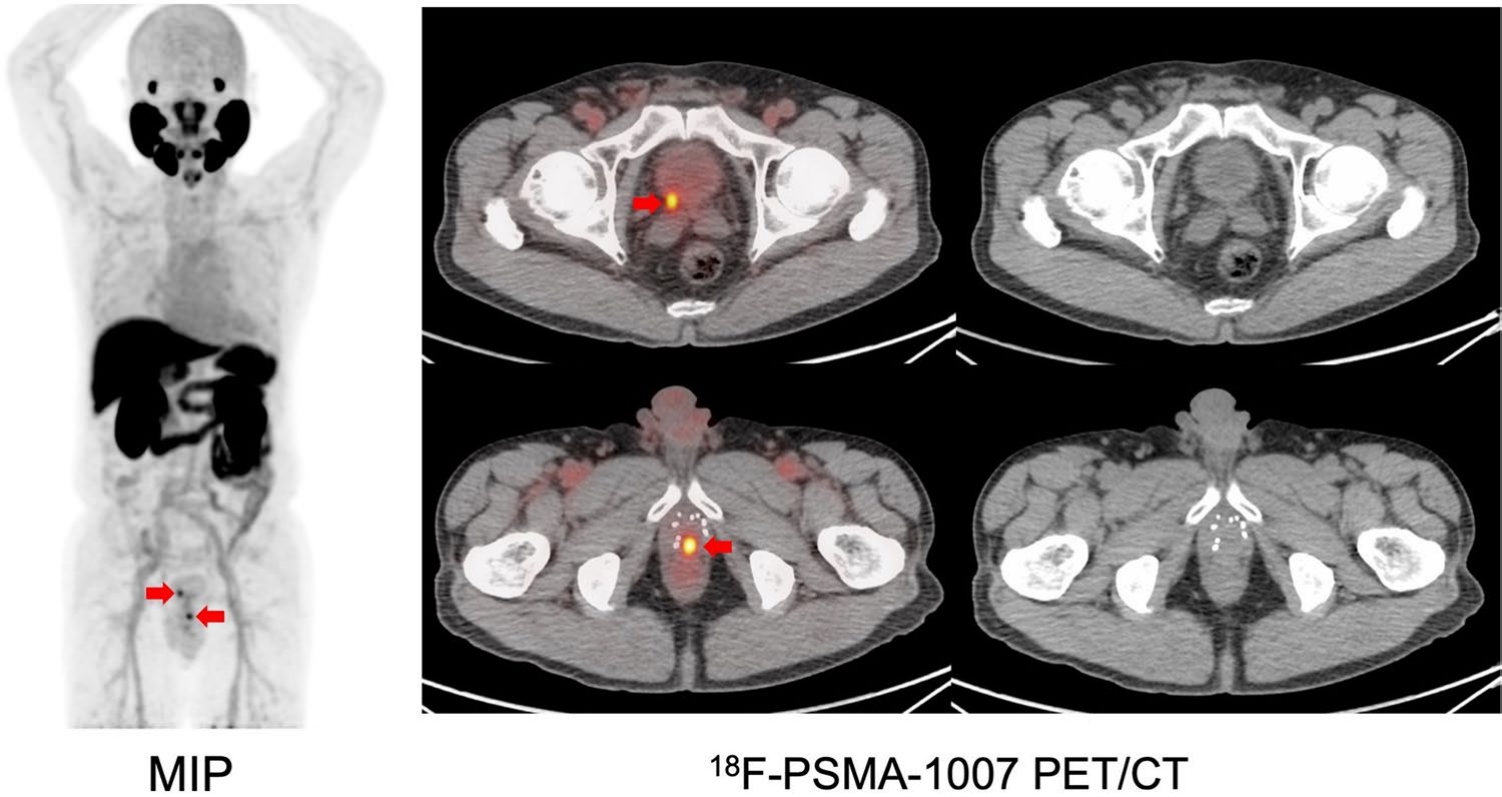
[^18^F]PSMA-1007 PET in BCR patient after ^125^I-seed implantation (PSA at PET: 3.32 ng/mL) (MIP: maximum intensity projection). Focal uptakes are observed on PSMA-PET (red arrows) and biopsy on the caudal lesion revealed a recurrence. Radiation therapy (cyber-knife) is performed targeting the two lesions and PSA value shows a decrease (PSA at 7 months after cyber-knife: 0.61 ng/mL)
